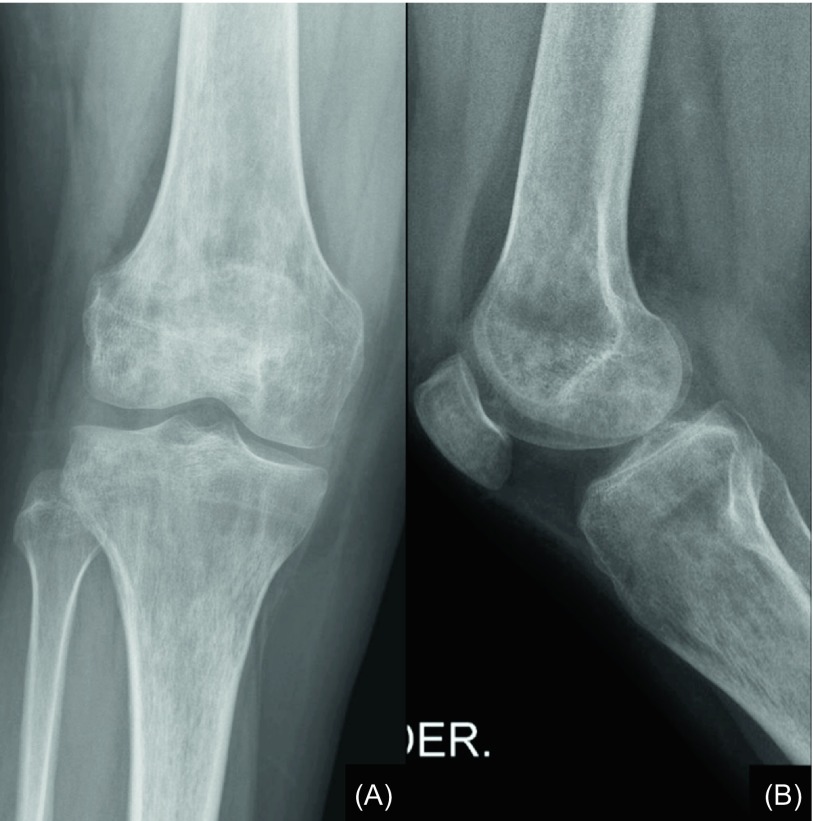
(A) Anteroposterior and (B) lateral radiographs of the patient mentioned on Figure 3 at a one-month period after the performance of the reimplantation, presenting mottled osteoporotic changes all along the distal femur and proximal tibia.
